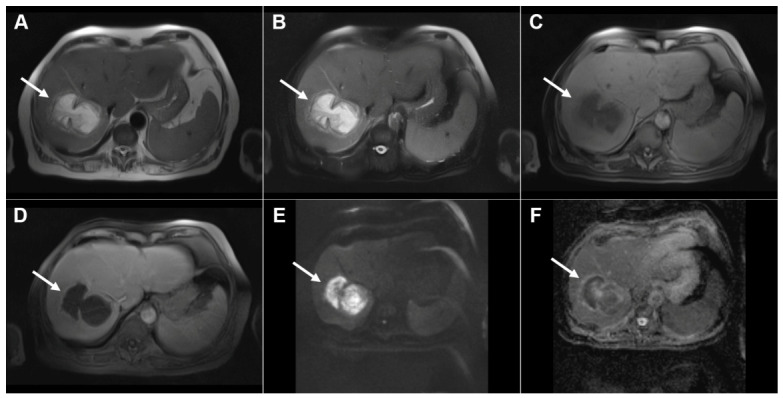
Images of a 79-year-old man with hepatic abscess. Axial T2-weighted (A) and T2-weighted fat-suppressed images (B) demonstrate a well-defined, lobulated cystic lesion (*arrow*) in liver segment VII with a uniformly thickened wall. Arterial (C) and portovenous phase (D) images show progressive rim enhancement. Marked diffusion restriction (E) is present within the lesion (*arrow*), with a corresponding low signal intensity on the ADC map (F), consistent with a hepatic abscess.
